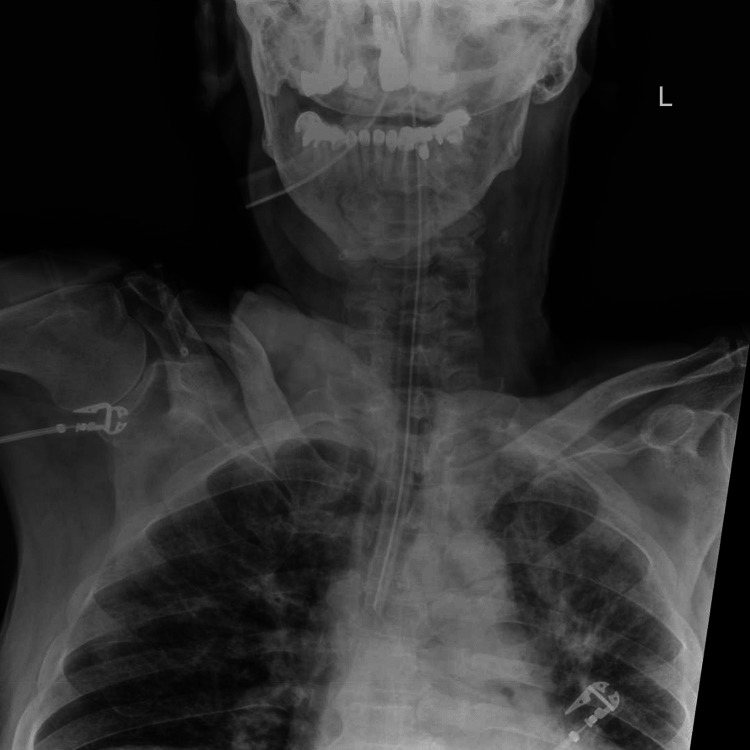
Antero-posterior (AP) radiograph of the neck and upper chest. This radiograph was obtained to localize a dislodged radiopaque tooth. No solitary tooth is identified in the mouth, neck, or upper chest.
